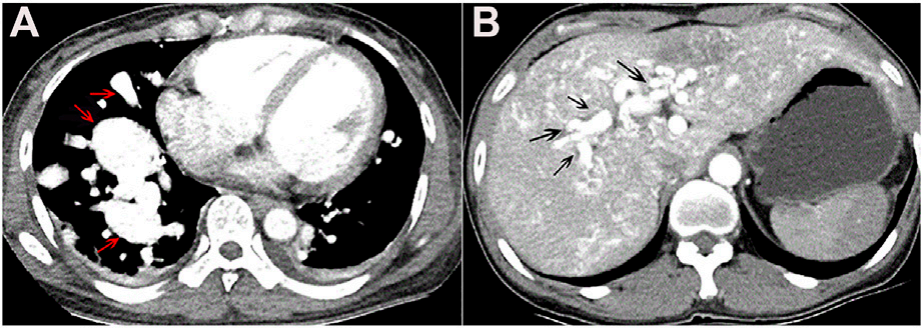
(A) Chest-enhanced CT image in the arterial phase showed multiple vascular masses in bilateral lungs, mainly in the lower lobe of the right lung, suggesting pulmonary arteriovenous fistula (red arrow). (B) Epigastrium-enhanced CT showed multiple hepatic arteriovenous malformations (black arrow).
